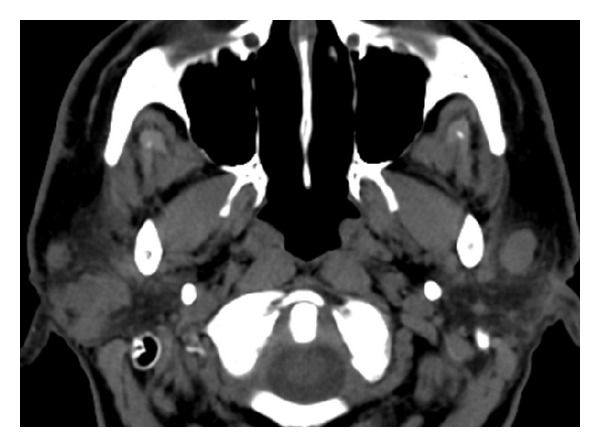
Head CT, noncontrast: multiple nodular masses of varying sizes in both parotid glands.
